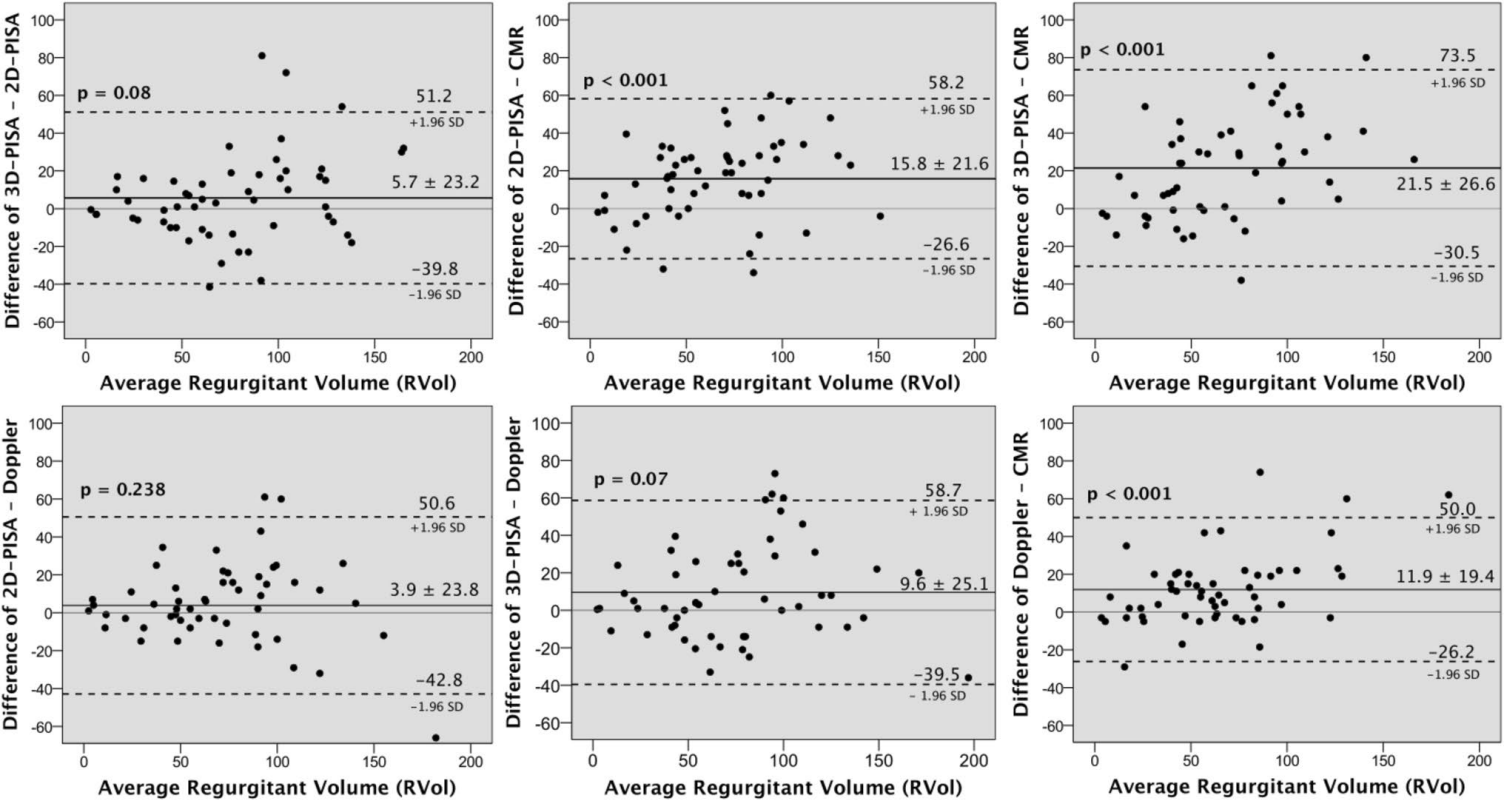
Bland–Altman plots for the agreement of measurements of regurgitant volume (RVol) by 2D-echocardiographic flow convergence method (2D-PISA), pulse-wave Doppler volumetric method (Doppler), RT-3DE derived PISA method (3D-PISA), and cardiac magnetic resonance (CMR), in patients with mitral valve prolapse
